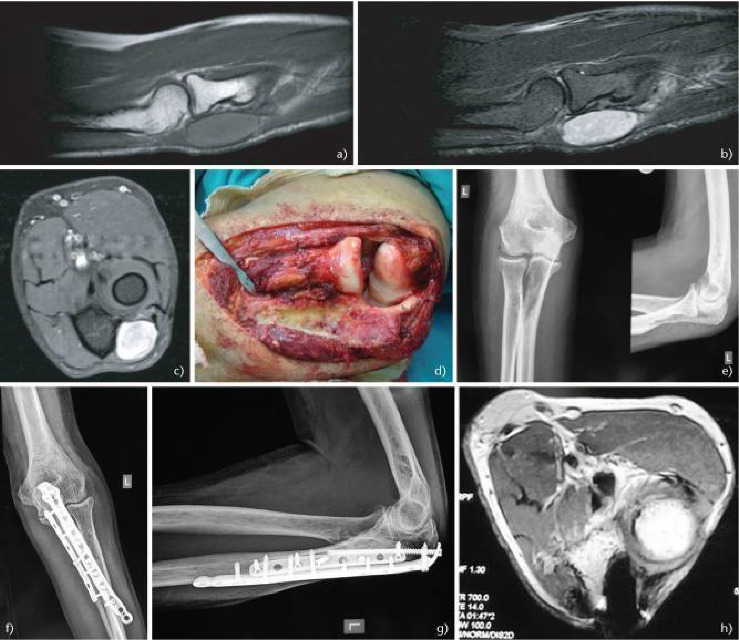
(a) Sagittal T1-weighted magnetic shows a mass with homogeneous intensity. (b) Sagittal T2 short tau inversion recovery (STIR). (c) Axial T1 fat saturated after contrast medium shows homogeneous enhancement of the lesion. (d) Intraoperative image after the wide excision of the synovial sarcoma. (e) Postoperative radiograph shows a pathological fracture in the proximal ulna due to radiation therapy and (f; g) its treatment after fixation with plates and screws. (h) Postoperative axial T1-weighted imaging after contrast medium shows no signs of recurrence 3 years after surgery.
